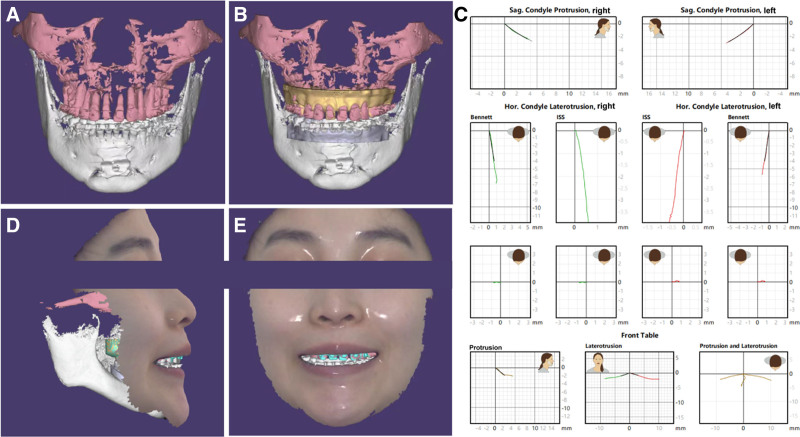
Prosthesis design. (A) CBCT, (B) intraoral scans, (C) digital cross-articulations, (D) facial scans, (E) 3D virtual patient.
